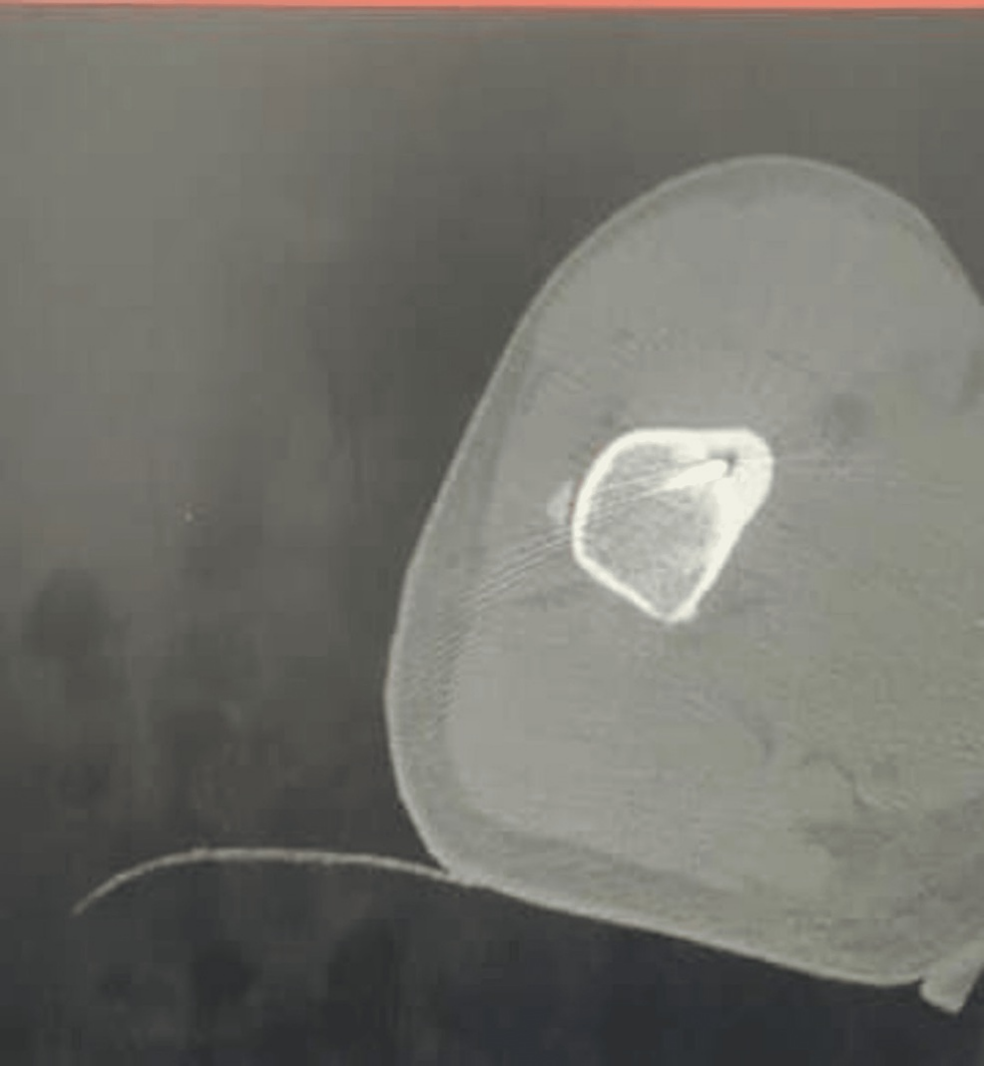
Axial section of multidetector computed tomography showing the radio frequency ablation probe inside the nidus
